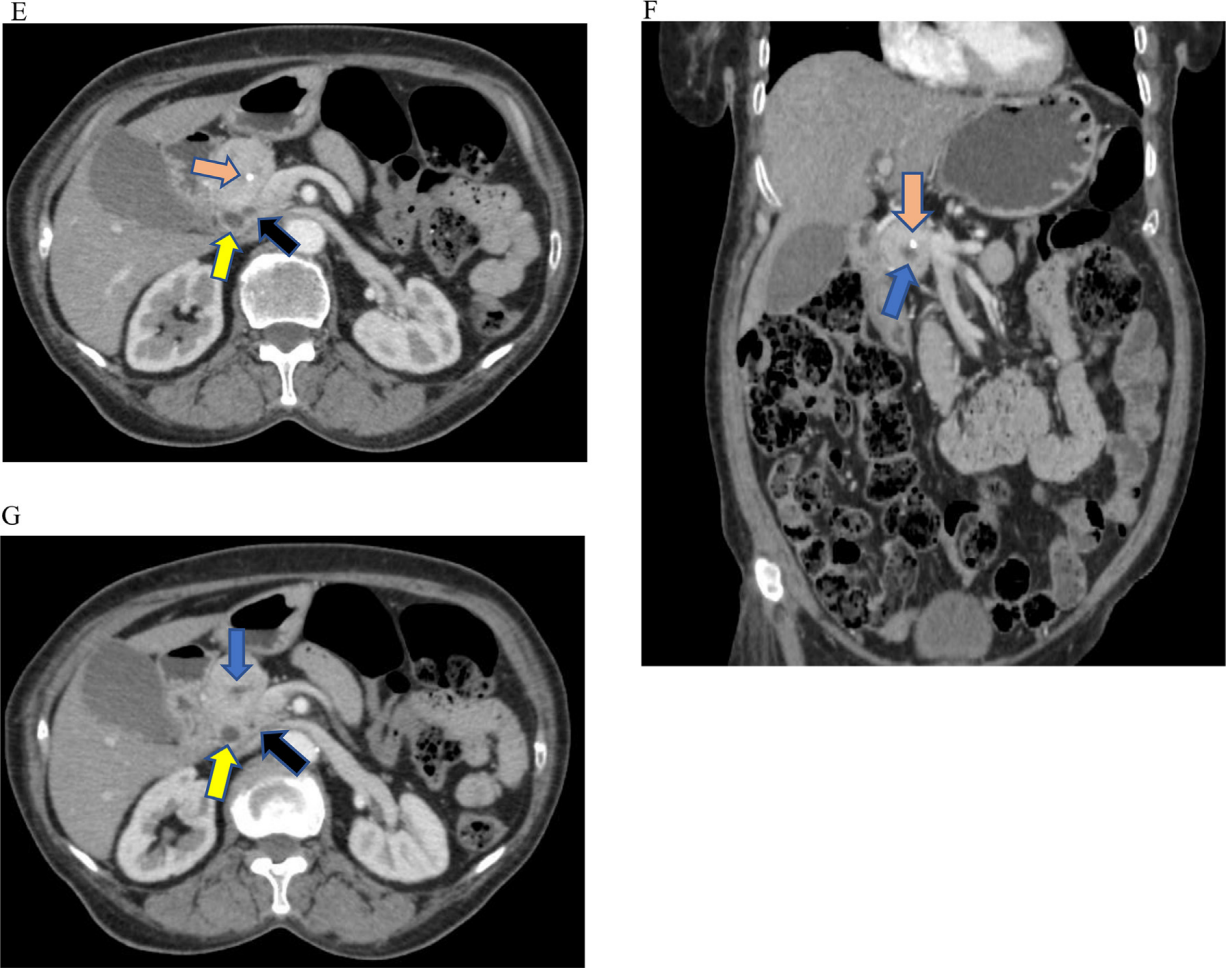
(A-G) Contrast-enhanced selected axial and coronal CT images of the abdomen demonstrating variable-sized calcifications at the uncinate/head of the pancreas (orange arrows) and an irregular cavity near the calcification (blue arrows). A portion of the ventral pancreas (red arrow) is appreciated. The retroperitoneal fat (green arrow) occupies the anatomic location of the dorsal pancreas. The Wirsung (black arrow) and Santorini ducts (white arrow) can be visualized clearly. Anatomic structures including the common bile duct (yellow arrow), duodenum (blue asterisk), and portal vein (purple arrow) are visible.
